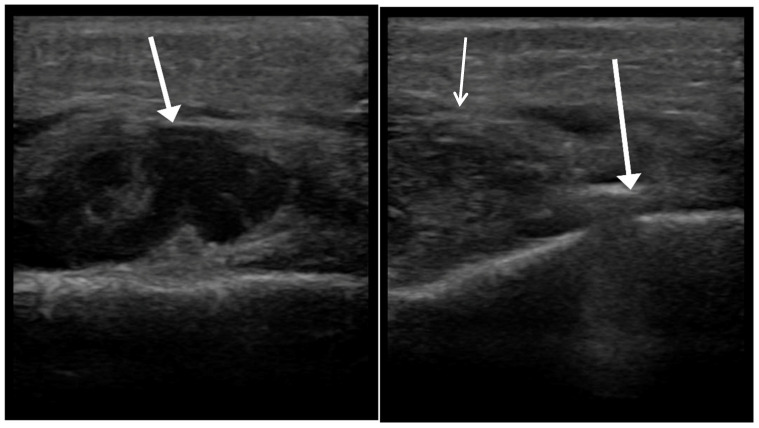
Handheld ultrasound imaging. Left: assessment of suspected abscess (arrow). Right: intraoperative ultrasound guidance confirming abscess location adjacent to periosteal elevator (arrow).
